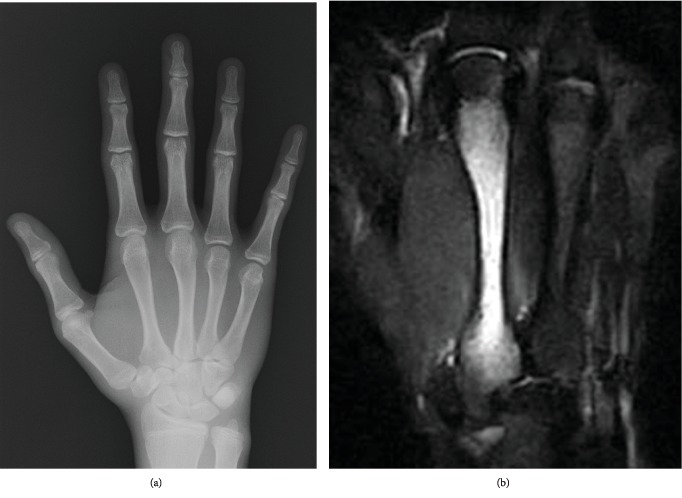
(a) Left hand radiograph showing no periosteal reaction or fracture line in the metacarpal. (b) Fat-suppressed fast spin-echo T2-weighted magnetic resonance showing high signal intensity in the shaft of the left third metacarpal.
